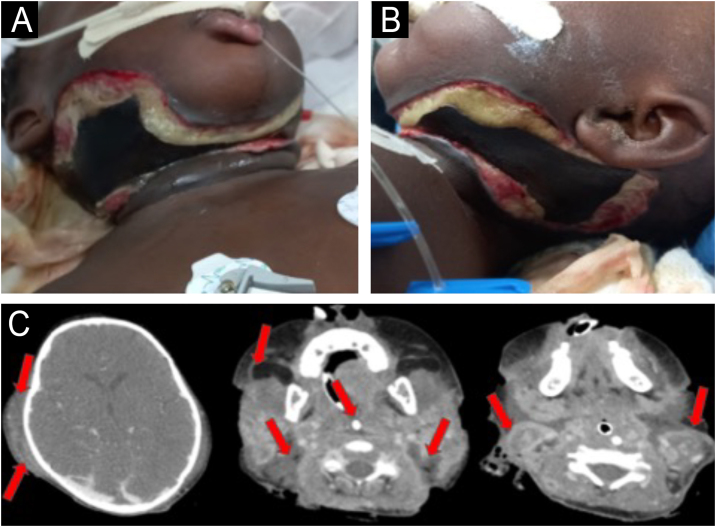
Extensive necrotic ulcer on the neck (A) right and anterior view (B) left view (C) CT scan showing thickening and densification of the myoadipose cervical planes and fascial enhancement (arrows).
